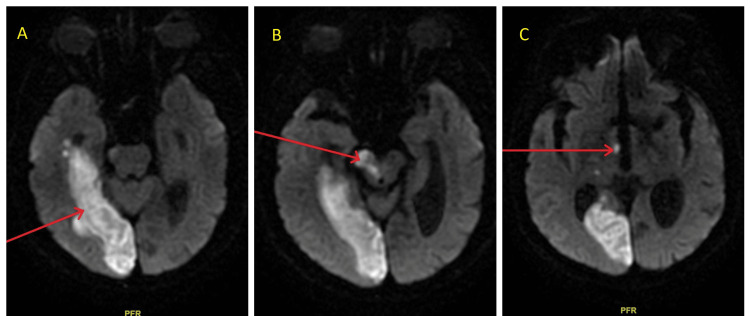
MRI brain without contrast Images (A)-(C) show acute or early subacute infarction (arrows) in the right posterior cerebral artery (PCA) territory, involving the medial temporal (A) and occipital lobes (A), as well as the midbrain (B) and the right thalamus (C). MRI: magnetic resonance imaging
